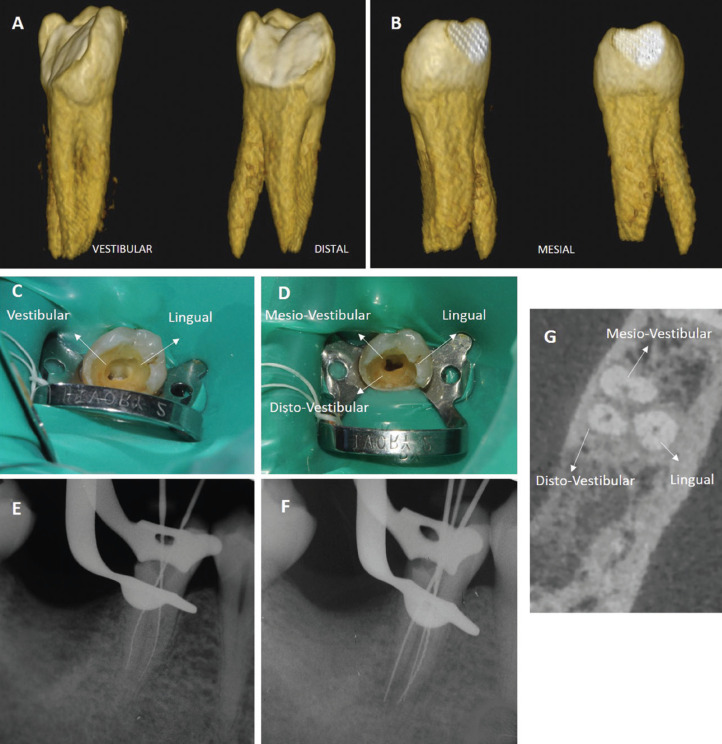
(A) Three-dimensional reproduction of the mandibular second premolar, seen from vestibular and distal. (B) Three-dimensional reproduction of the mandibular second premolar seen from mesial. (C) Cameral opening with the location of two canals. (D) Cameral opening with the location of three canals. (E) Periapical radiograph with the location of two roots and two canals. (F) Periapical radiograph with the location of the three canals and the three roots. (G) CBCT image with the three roots and the three canals.
